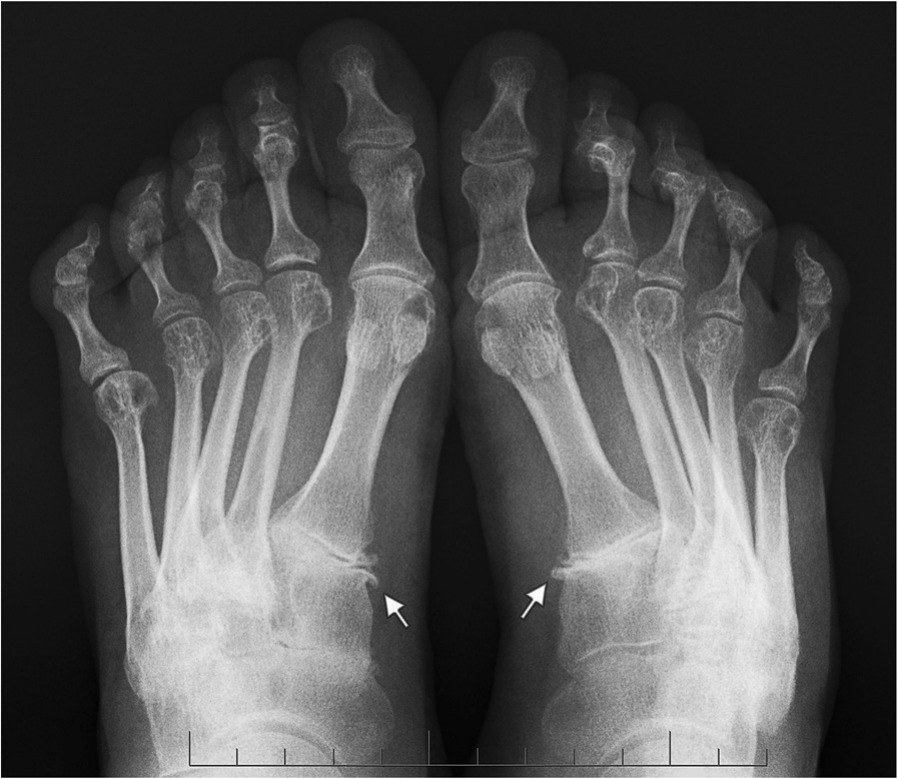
Sign of the metatarsophalangeal instability. Anteroposterior X-ray of a 40 year old woman with rheumatoid arthritis shows signs of the metatarsophalangeal instability, osteophytes of both medial cuneometatarsal joints (white arrows).
